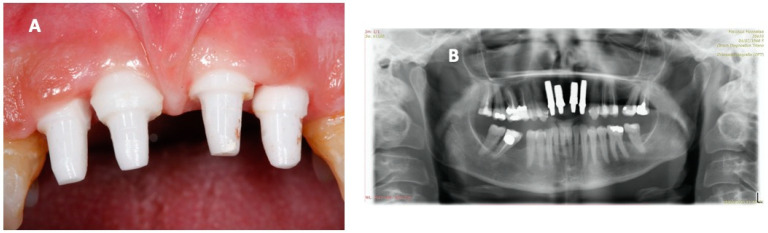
Pictures obtained six months after the operation, showing (A) a good state of osteointegration, no bleeding, and no active infection, with healthy periodontal tissue, and (B) dental radiography showing a good grade of osteointegration and no significant radiolucent areas.
